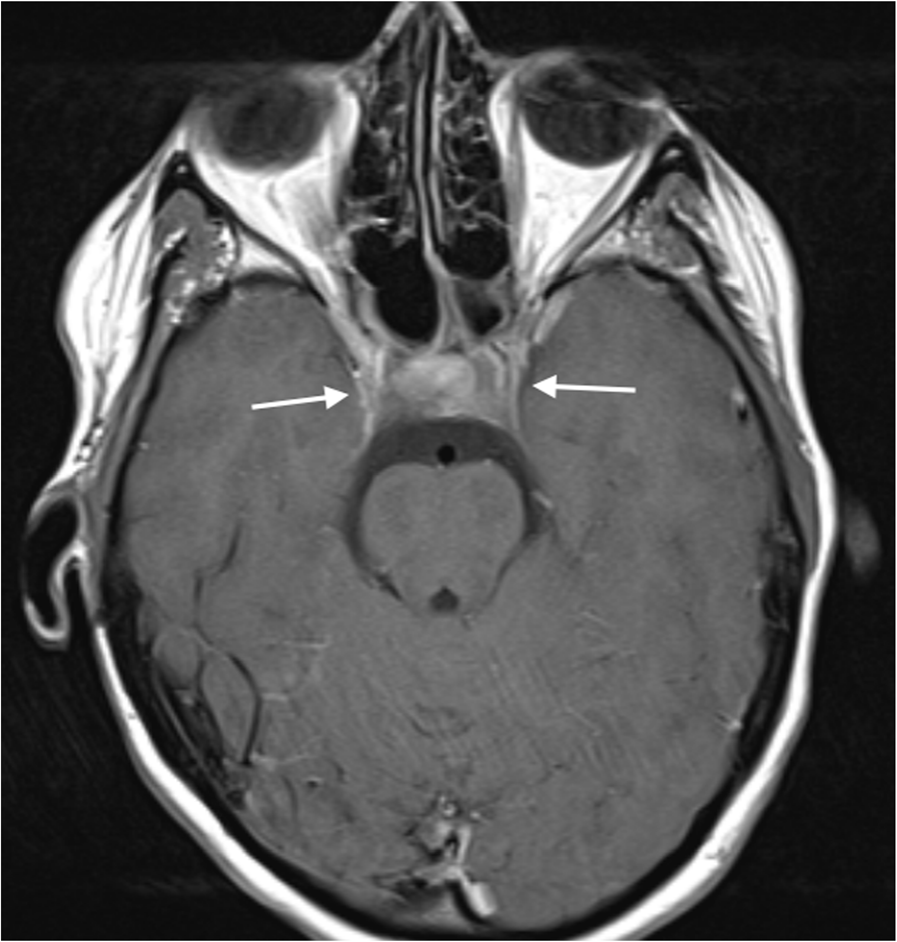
Axial intracranial contrast-enhanced T1-weighted MR image after six pulses of cyclophosphamide. There is reduction in the volume of abnormal cavernous sinus enhancing chronic inflammatory tissue (arrows) compared with pre-treatment imaging
